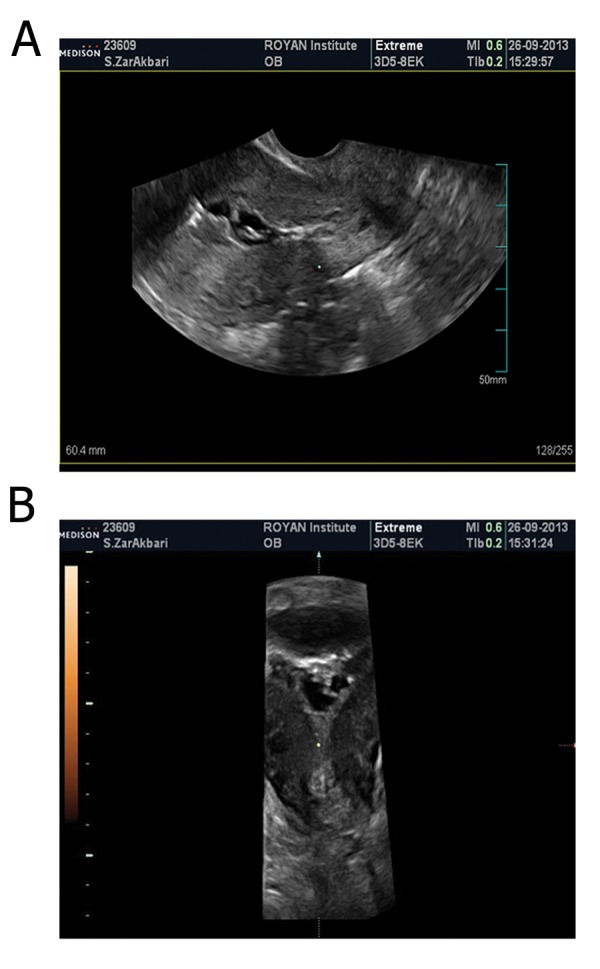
Sagital and coronal saline hysterosonogram showing
